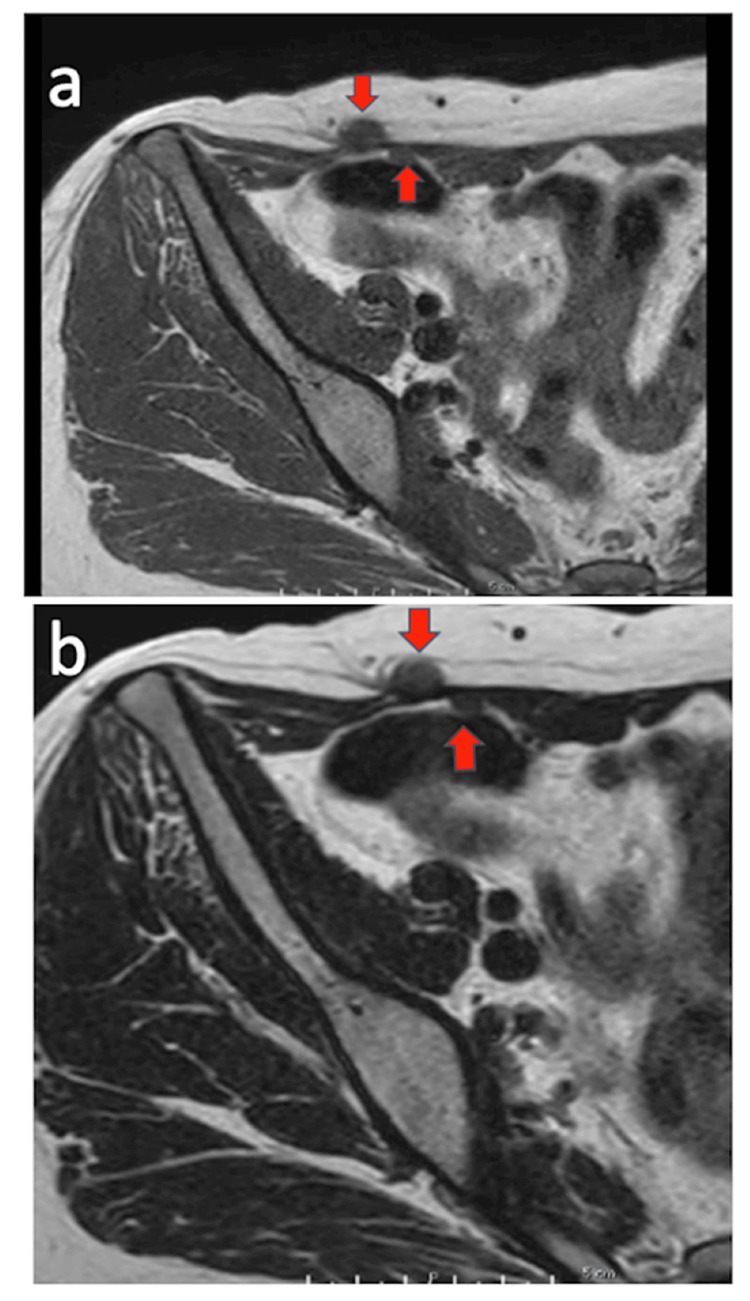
Magnetic resonance image (MRI) of the right abdomen. (a) T1-weighted axial image. (b) T2-weighted axial image. Arrows show the position of masses.
